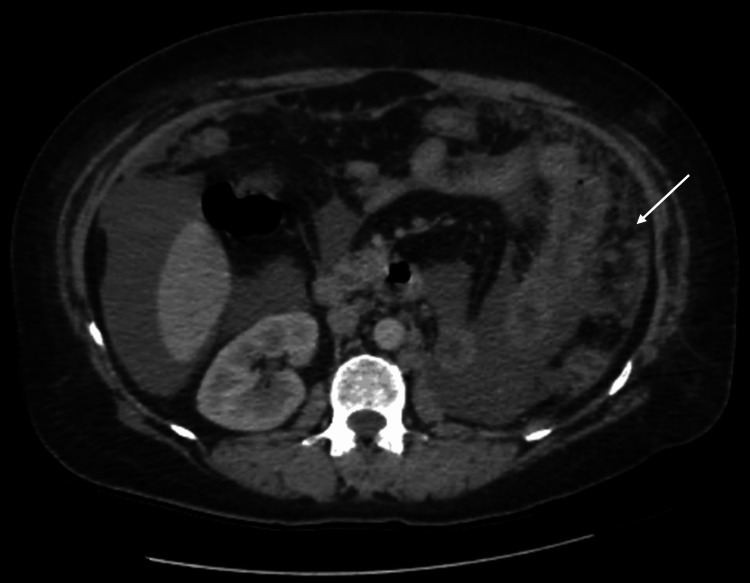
Axial CT scan showing a stranding pattern of the peritoneal fat consistent with peritoneal carcinomatosis
